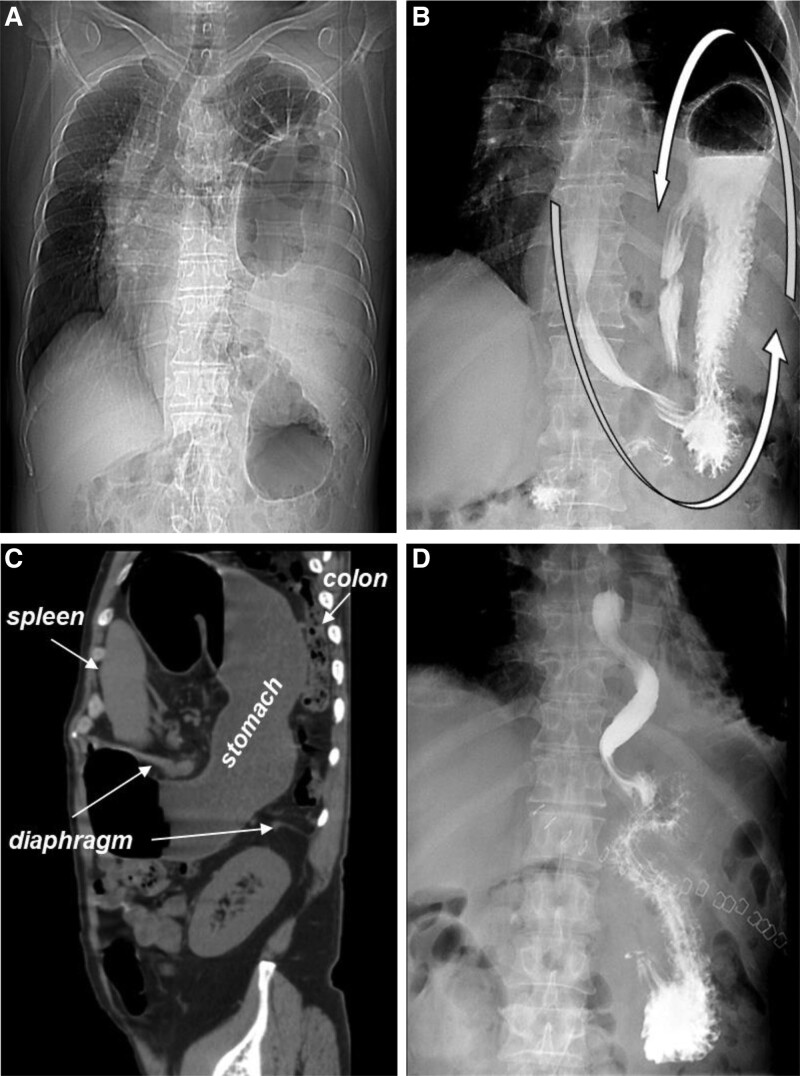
(A) Chest radiograph shows elevated left diaphragmatic dome with rightward shift of mediastinum. (B) Upper gastrointestinal barium study shows stomach was upside-down. (C) Abdominal CT reconstructed sagittal image shows stomach, spleen, and colon passing through left hemithorax via large diaphragmatic defect. (D) Postoperative barium meal imaging indicates stomach’s return to normal position.
